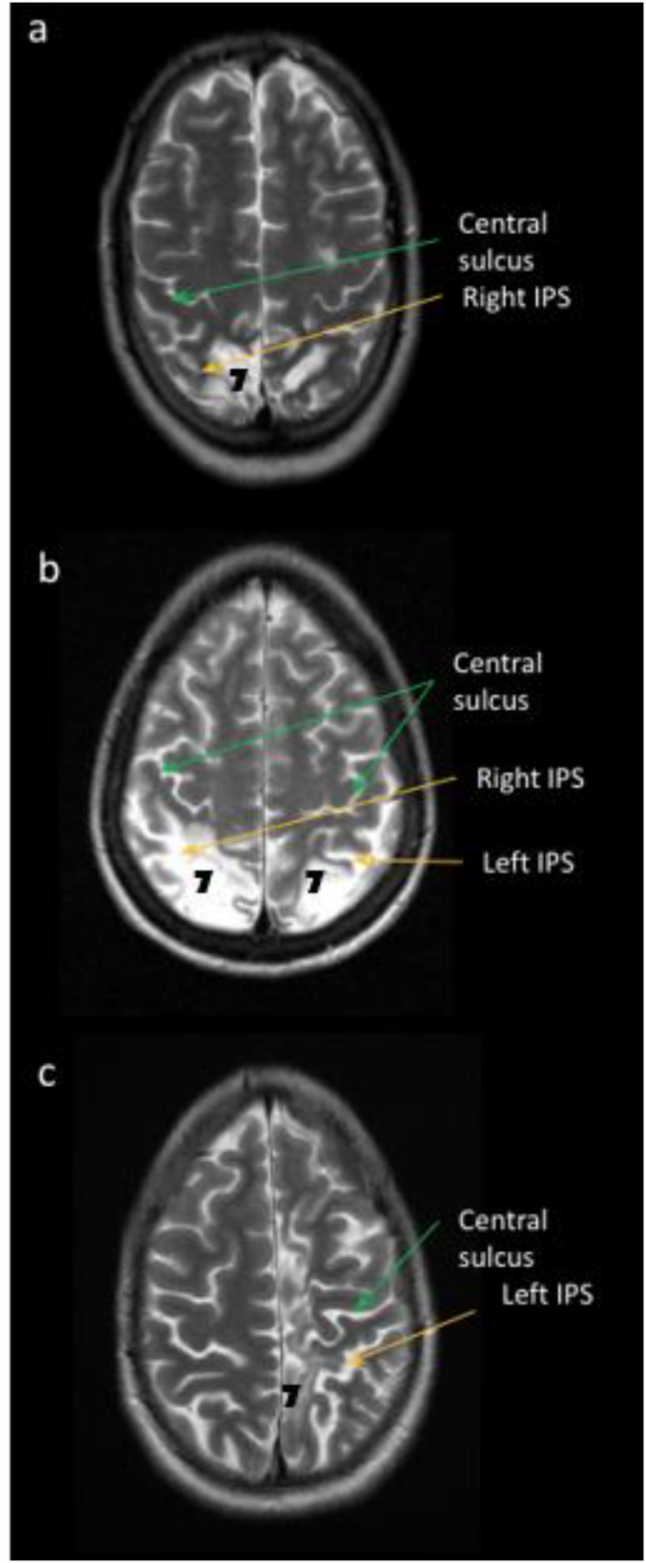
MRI horizontal T2-weighted images of optic ataxia patients. Patient CF (a), patient IG (b), and patient MO (c) display lesion of BA 7 (SPL-IPS region).
